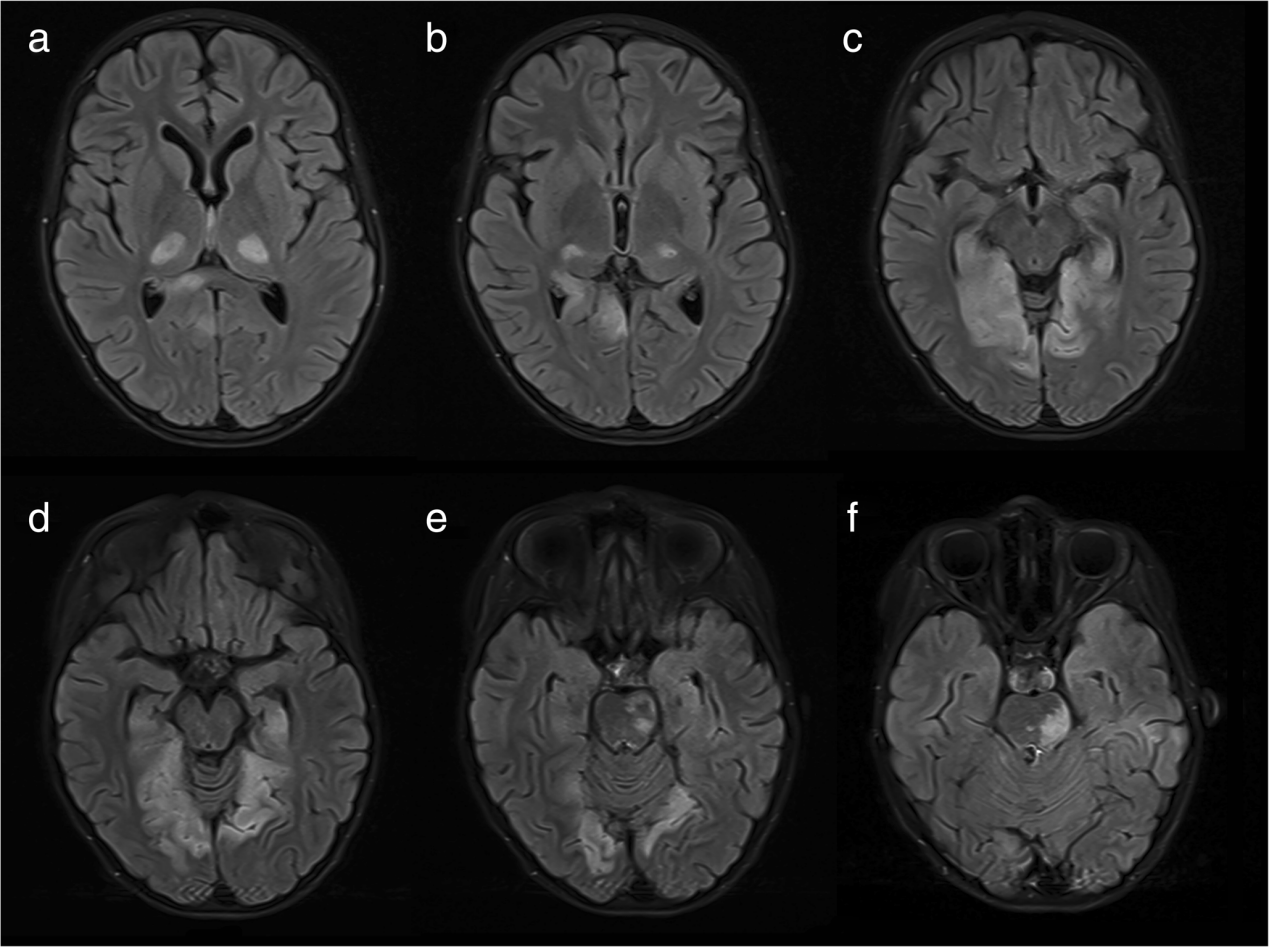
T2-weighted dark fluid MR scans show symmetrical hyperintense areas in both thalami (a, b) and medial parts of occipital lobes (c, d, e). Note left-sided pontine hyperintensivity (e, f) and involvement of splenium of corpus callosum (a)
